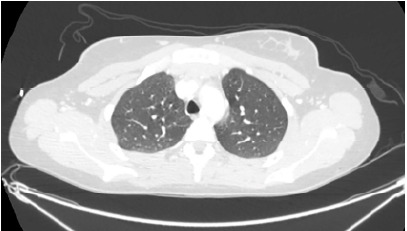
Outside hospital computed tomography chest showing no acute abnormalities.
